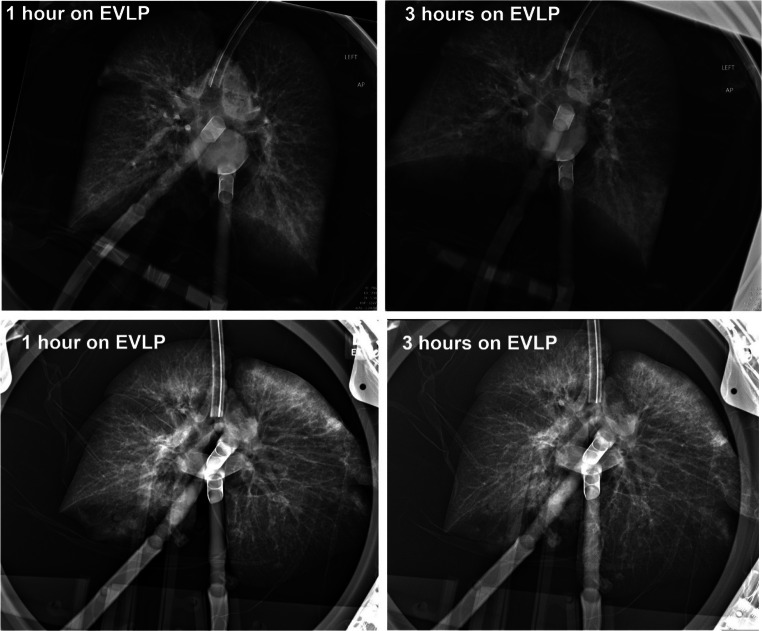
CXR of a successful EVLP run (CXR, chest X-ray; EVLP, ex vivo lung perfusion)
